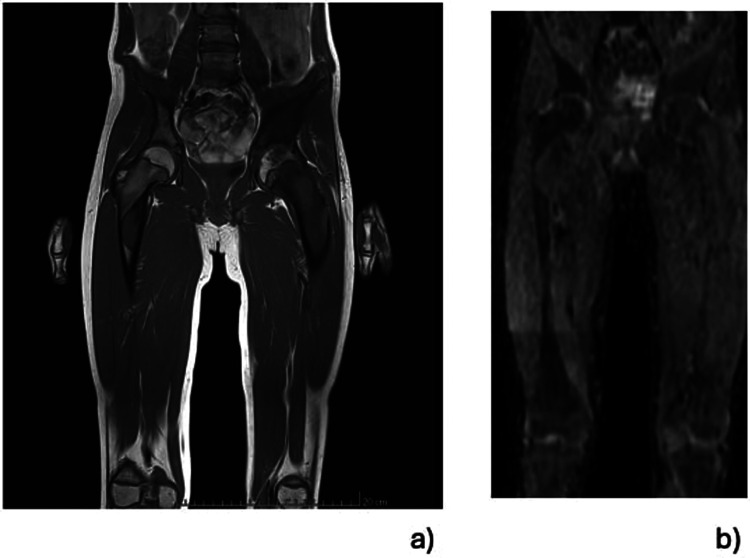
Selected total-body MRI at the level of the pelvis and both femurs using low signal intensity on T1-weighted images and demonstrating the foci of FD affecting the epiphysis, the femoral neck, the diaphyseal regions of the left femur and the same hip's acetabulum. A lesion is visible in the subtrochanteric region of the right femur (a). There was a high signal intensity without diffusion restriction on the fat-suppressed T2-weighted images enhanced with gadolinium (b).
